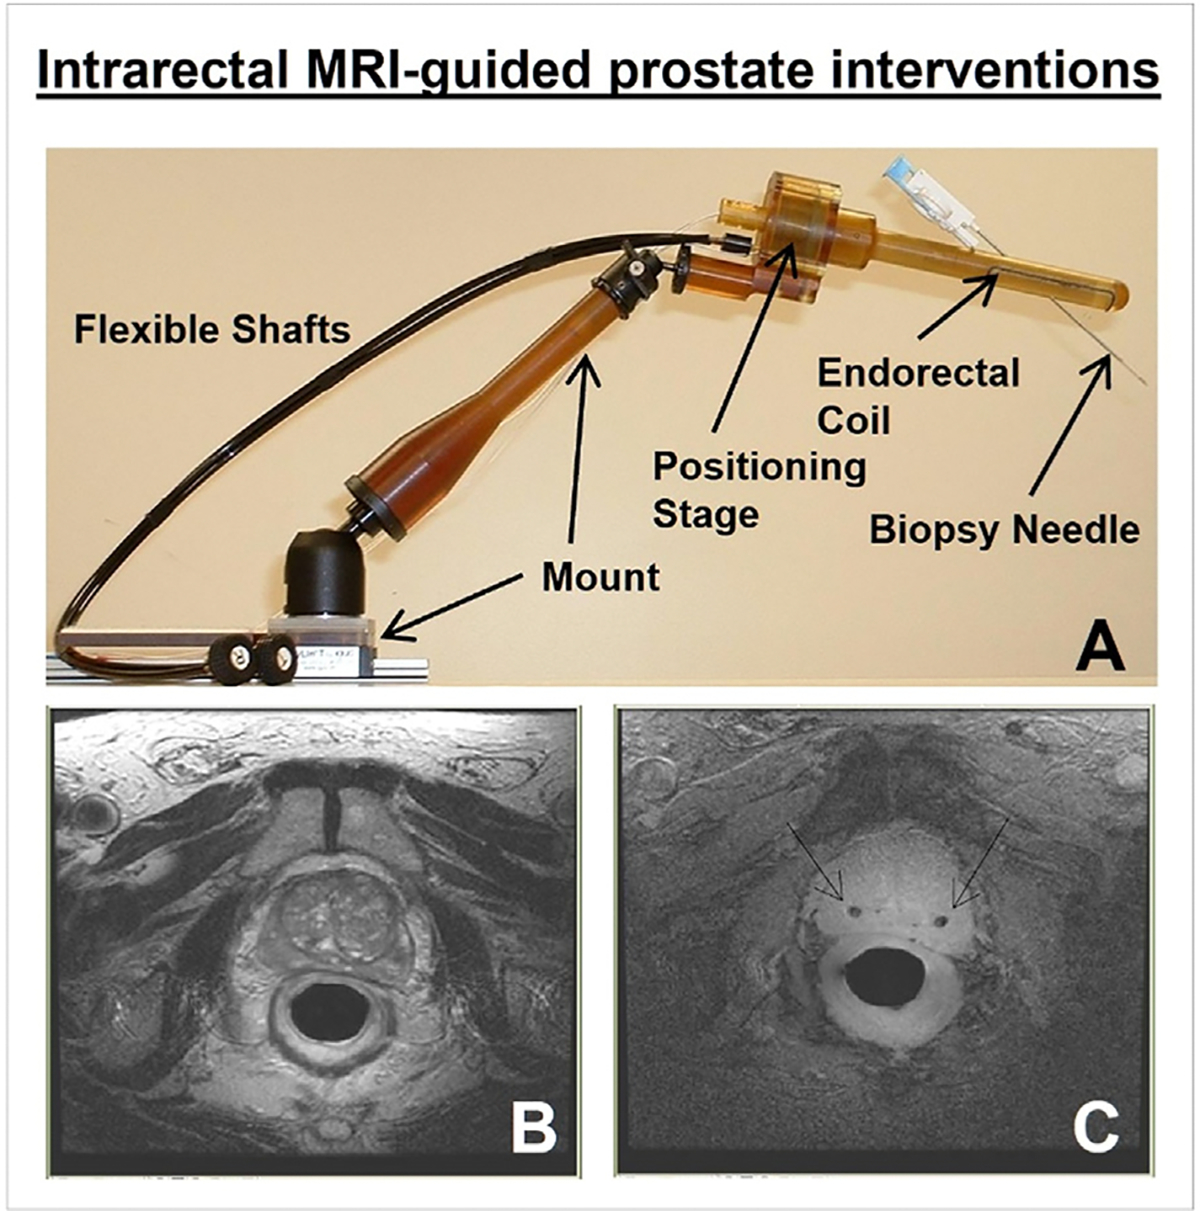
(A) A transrectal MRI device with a needle guide and sheath, positioning stage, insertion stage, flexible actuation shafts, and mount. (B) Transrectal cross-sectional high-resolution MRI of the prostate, using the manipulator for precise insertion of two radiation seeds (arrows in C) into the prostate for internal radiation therapy (Courtesy of Robert Susil).
